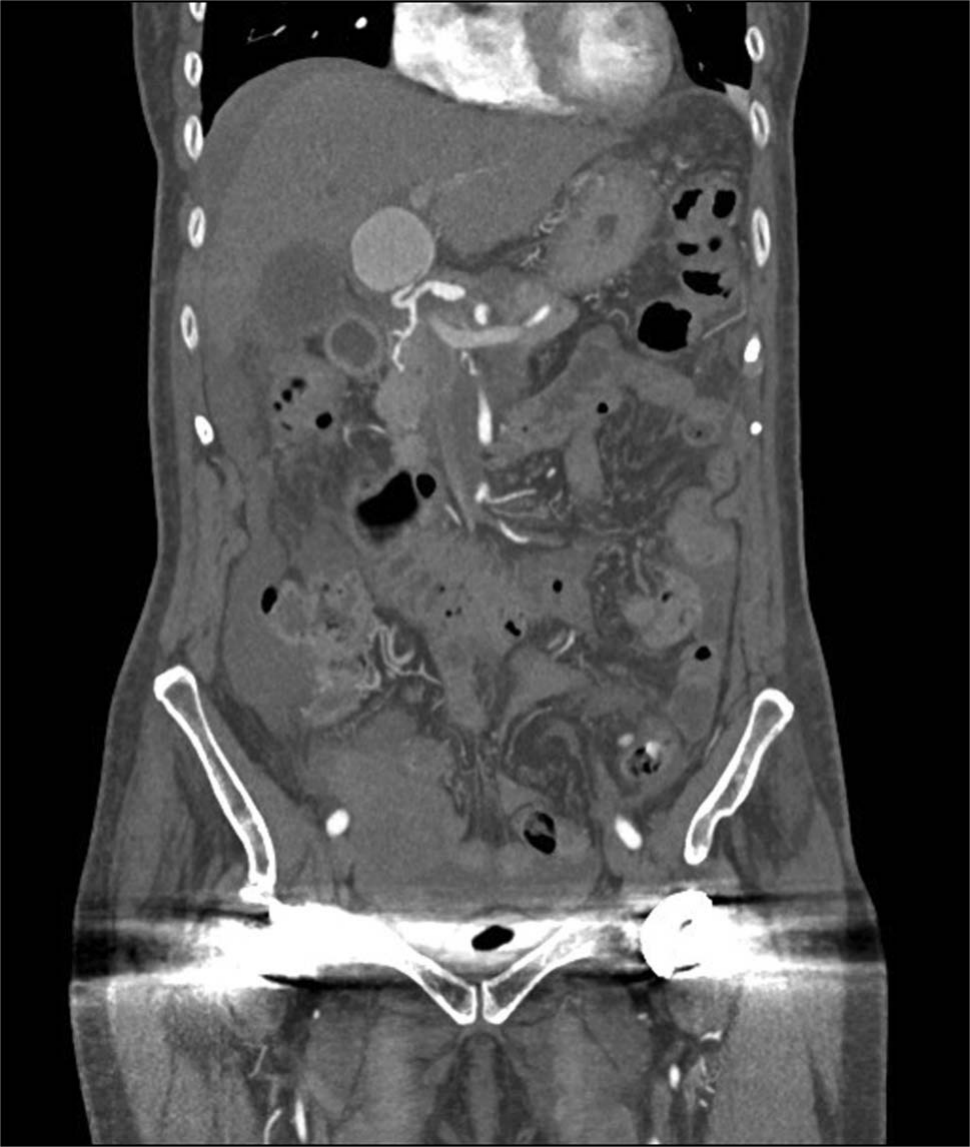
Coronal view of pre-embolization abdominal computed tomography with left hepatic artery pseudoaneurysm.
